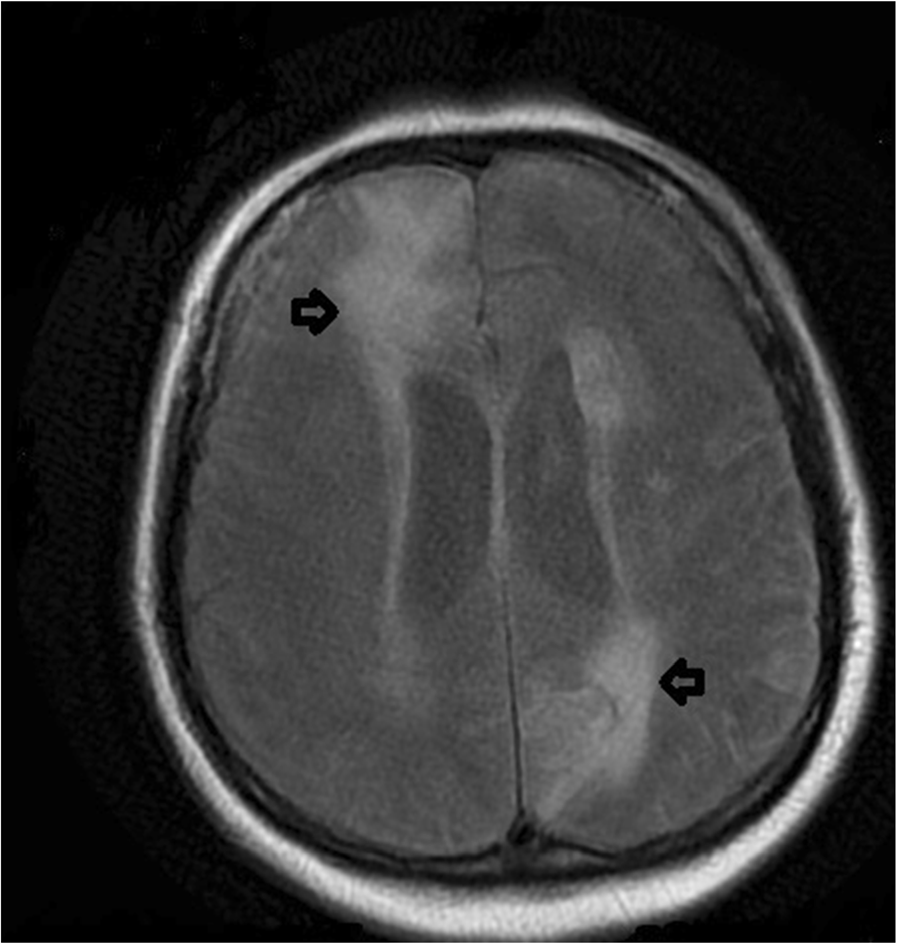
Diffusion Weighted Image-Magnetic Resonance Image. A Diffusion Weighted Magnetic Resonance Image showing multiple lesions with restricted diffusion (see arrows) consistent with multiple abscesses and developing hydrocephalus
